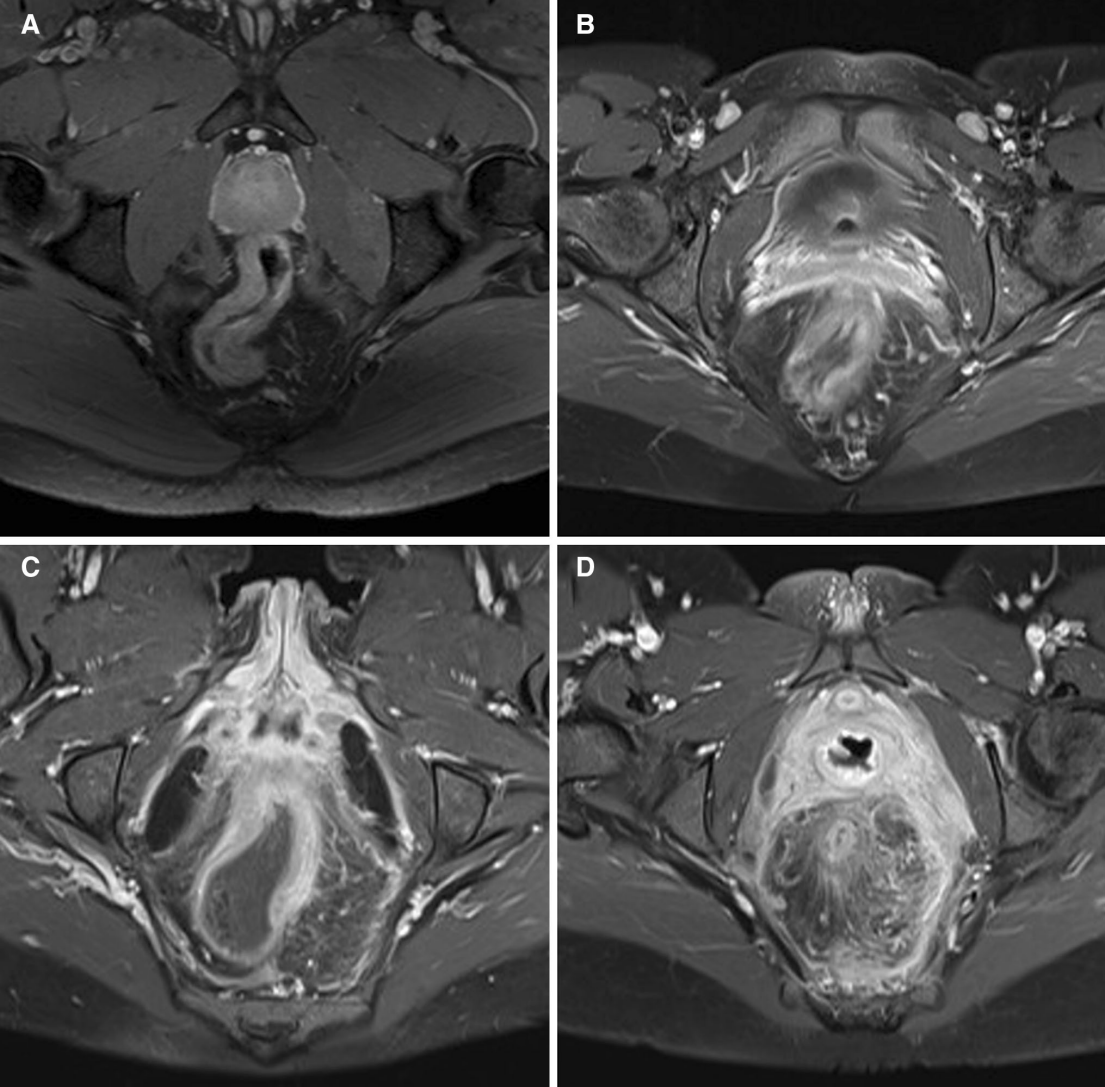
Axial oblique fat-saturated post-contrast T1-weighted images of four different patients with Crohn’s disease with different degrees of perimural enhancement. A Equivalent to normal fat tissue. B Minor enhancement. There is blurred demarcation of the bowel wall with or without mild increase of perimural fat tissue signal. C Moderate enhancement. Increase of perimural fat tissue signal but less than nearby vascular structures. D Marked enhancement. Perimural fat tissue signal approaches that of nearby vascular structures. Mesorectal fascia enhancement can be noted
